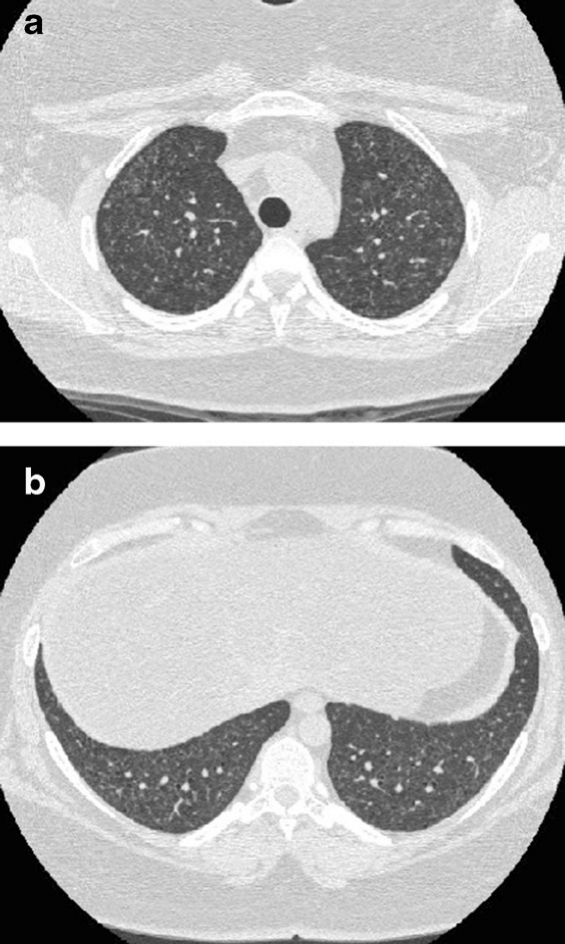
A 34-year-old woman non-smoker with PLCH symptomatic for dry cough. Definitive diagnosis obtained by VATS performed within a month of imaging studies. a, b HRCT images at diagnosis shows ill-defined micronodularity associated with predominant reticular and minimal bronchiectatic changes. No basal regions sparing
